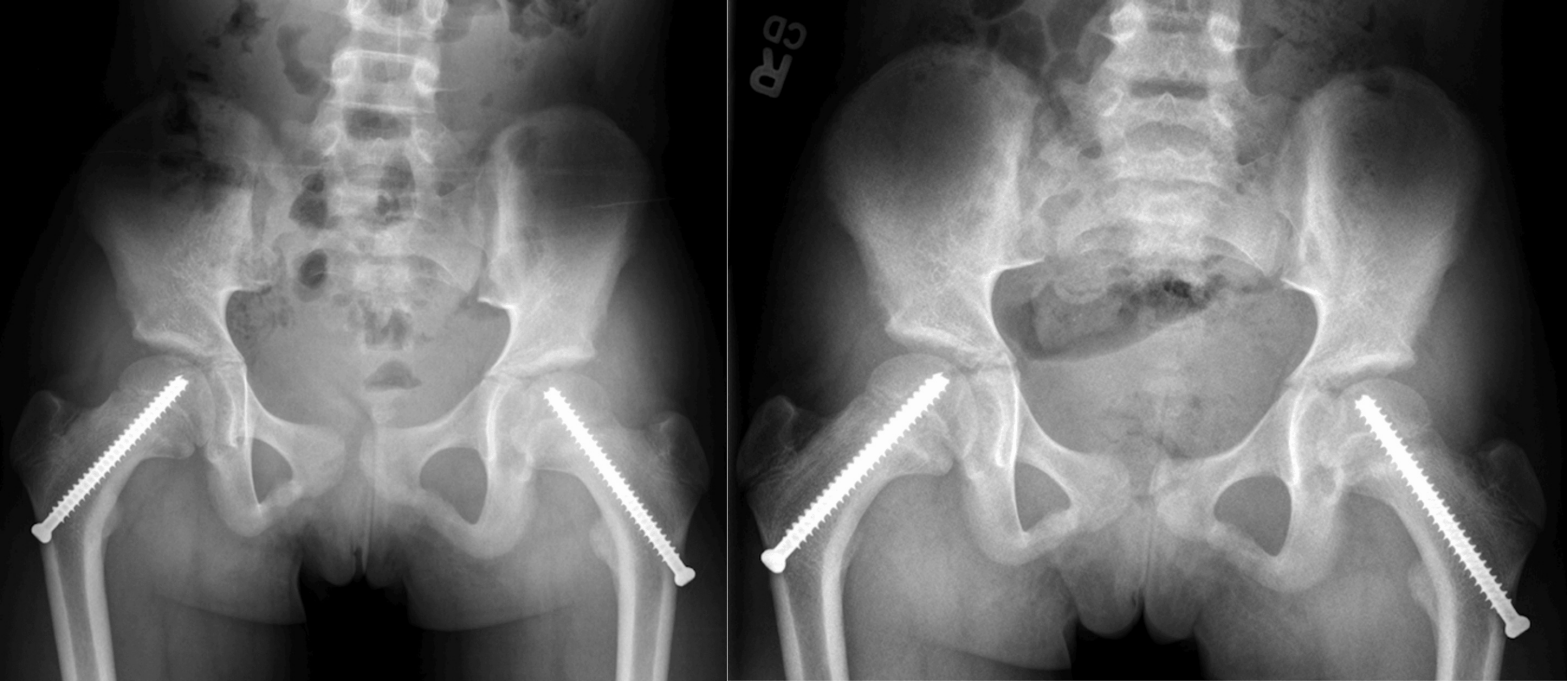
Anterioposterior and frog leg X-rays at the six-month follow-up after bilateral screw replacement. X-rays show adequate screw position and closing of growth plates.
